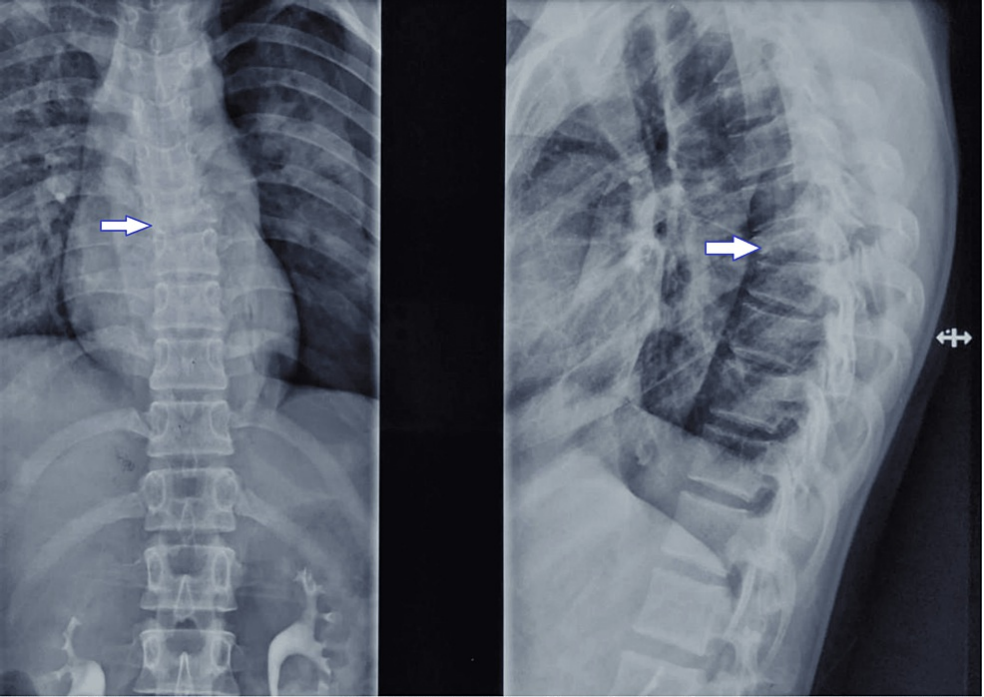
X-ray of vertebral injury – AP and lateral view AP, anteroposterior.
